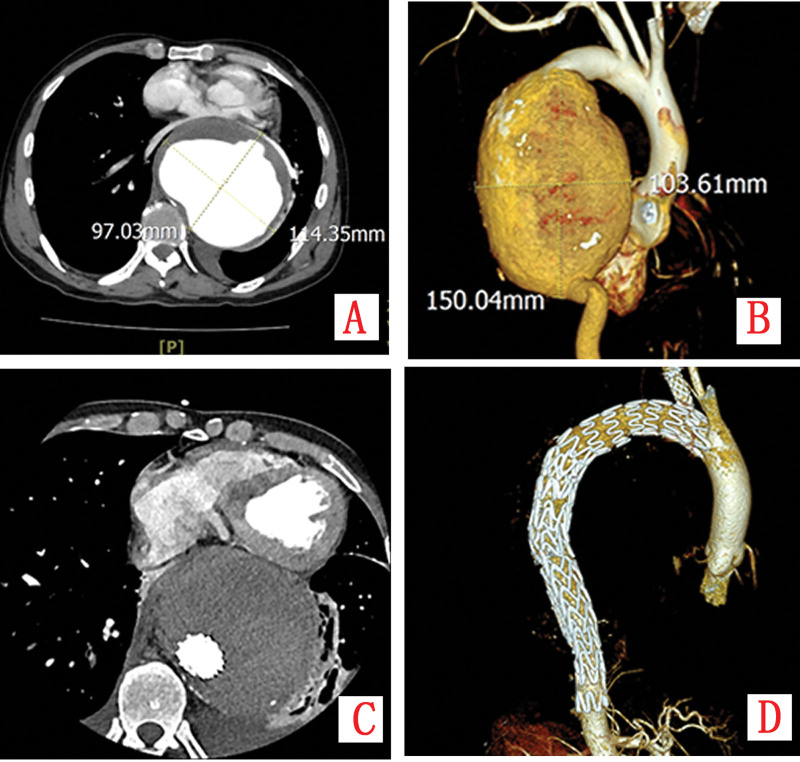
(A, B) CTA reveals a giant fusiform thoracic aortic aneurysm measuring 10.8 × 9.2 × 16.1 cm with peripheral calcification, compressing the esophagus, trachea, and left hilum, accompanied by minimal left pleural effusion without contrast extravasation; (C, D) post-TEVAR CTA demonstrates perigraft thrombosis and absence of endoleak.
